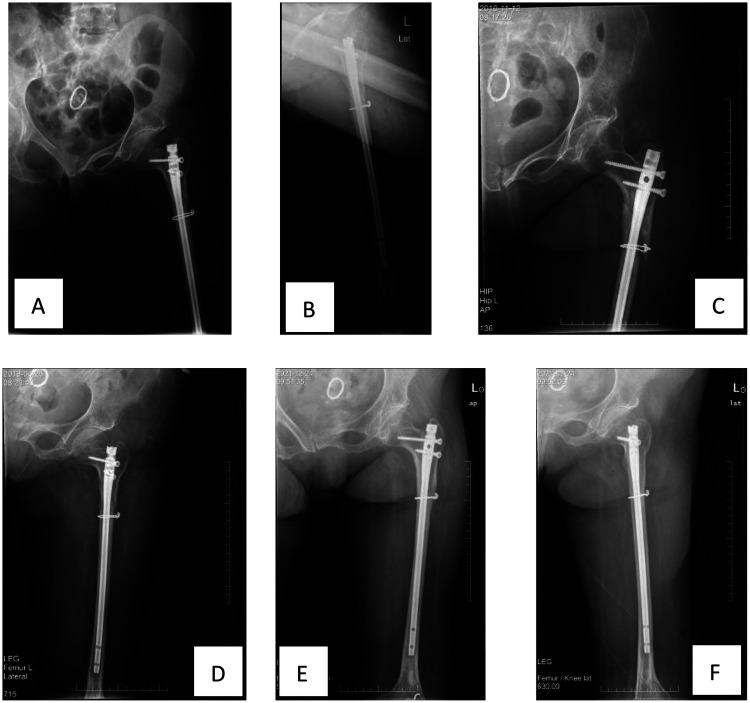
Postoperative follow-up: (A) anteroposterior x-ray on the third postoperative day. (B) Lateral x-ray film 3 days postoperatively. (C) Anteroposterior x-ray at 1 month postoperatively. (D) Anteroposterior x-ray at 5 months postoperatively. (E) Anteroposterior x-ray at 30 months postoperatively. F. Lateral x-ray at 30 months postoperatively.
